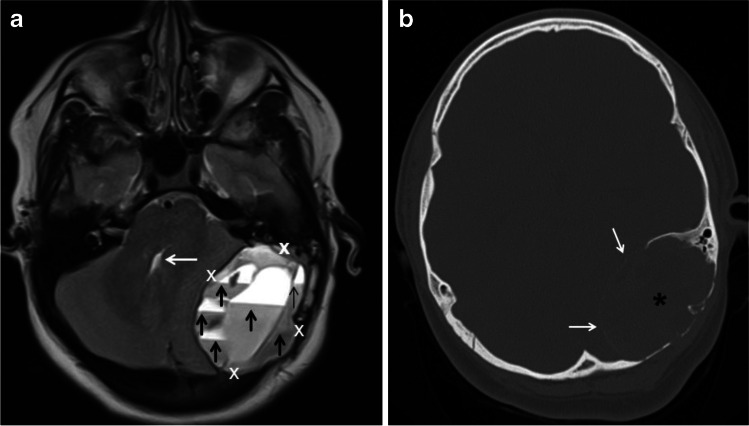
Aneurysmal bone cyst (ABC) of the skull and petrous bone in a 10-year-old boy. a An axial T2-weighted magnetic resonance image of the head shows a large, elliptical, expansile mass (calipers) in the left side of the posterior fossa causing mass effect upon the left cerebellar hemisphere and partial effacement of the fourth ventricle (*white arrow*). The mass is multiseptated and contains fluid-fluid levels of different signal intensity distributed throughout the lesion (*black arrows*). b An axial computed tomography scan image using bone windows shows to better advantage the petrous bone and occipital bone involvement by the ABC (*asterisk*) as well as a thin peripheral calcified shell (*white arrows*)
